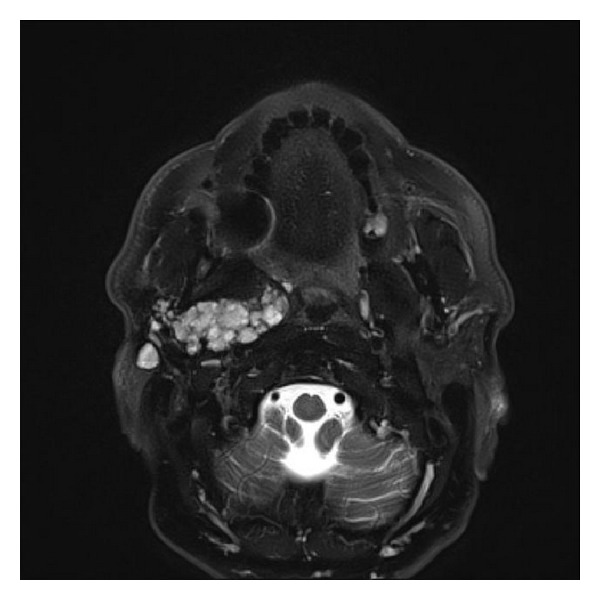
Preoperative MRI T2 Fat Sat.
